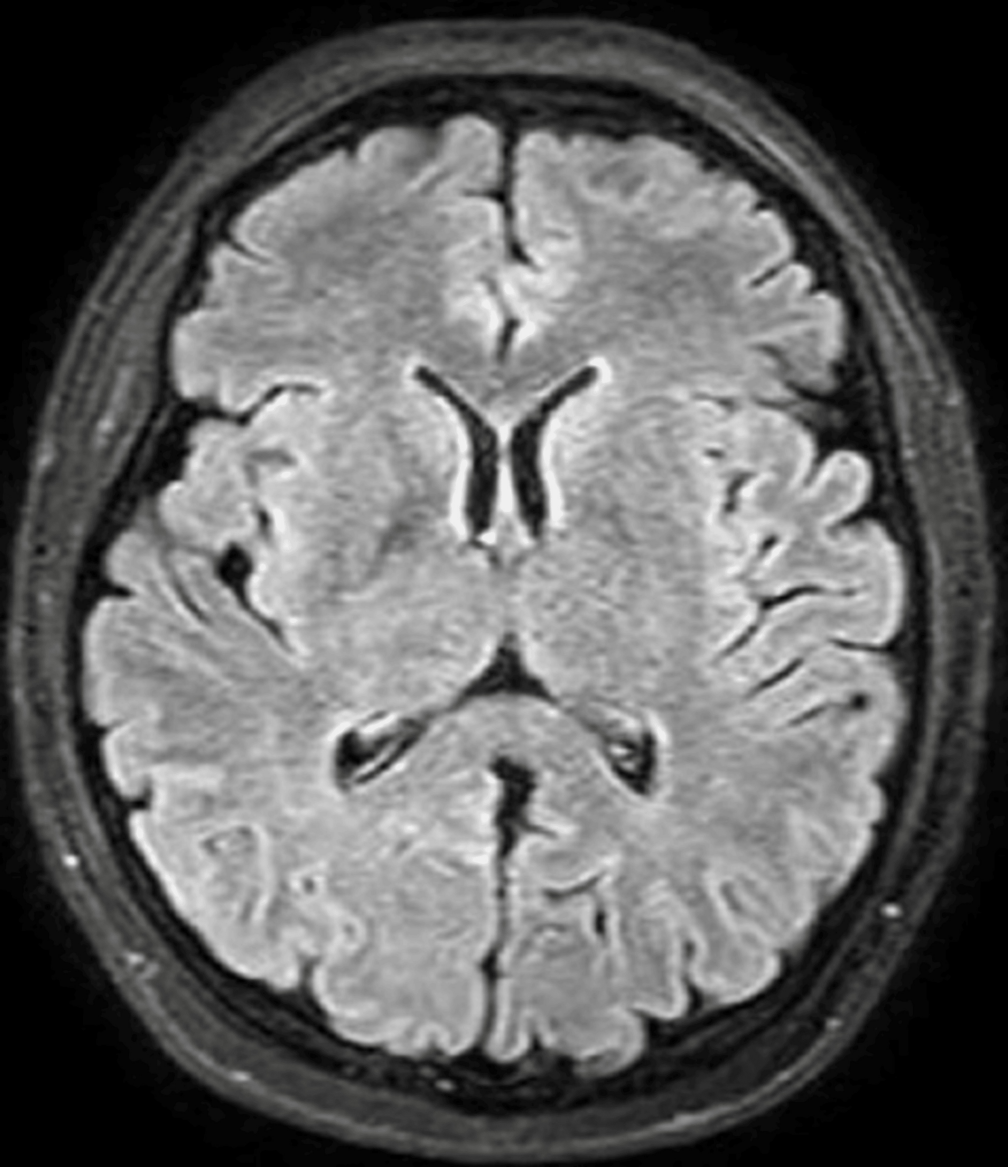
Magnetic resonance imaging (MRI) of the brain, taken on the post-operative day (POD) 1, revealed no signs of acute abnormalities.
